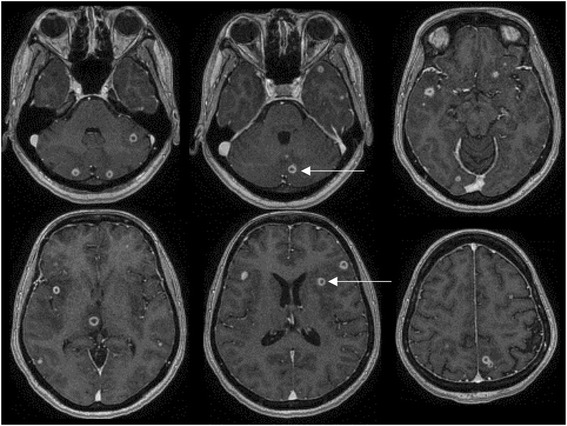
Maternal MRI showing small ring-enhancing lesions in both cerebral and cerebellar hemispheres
